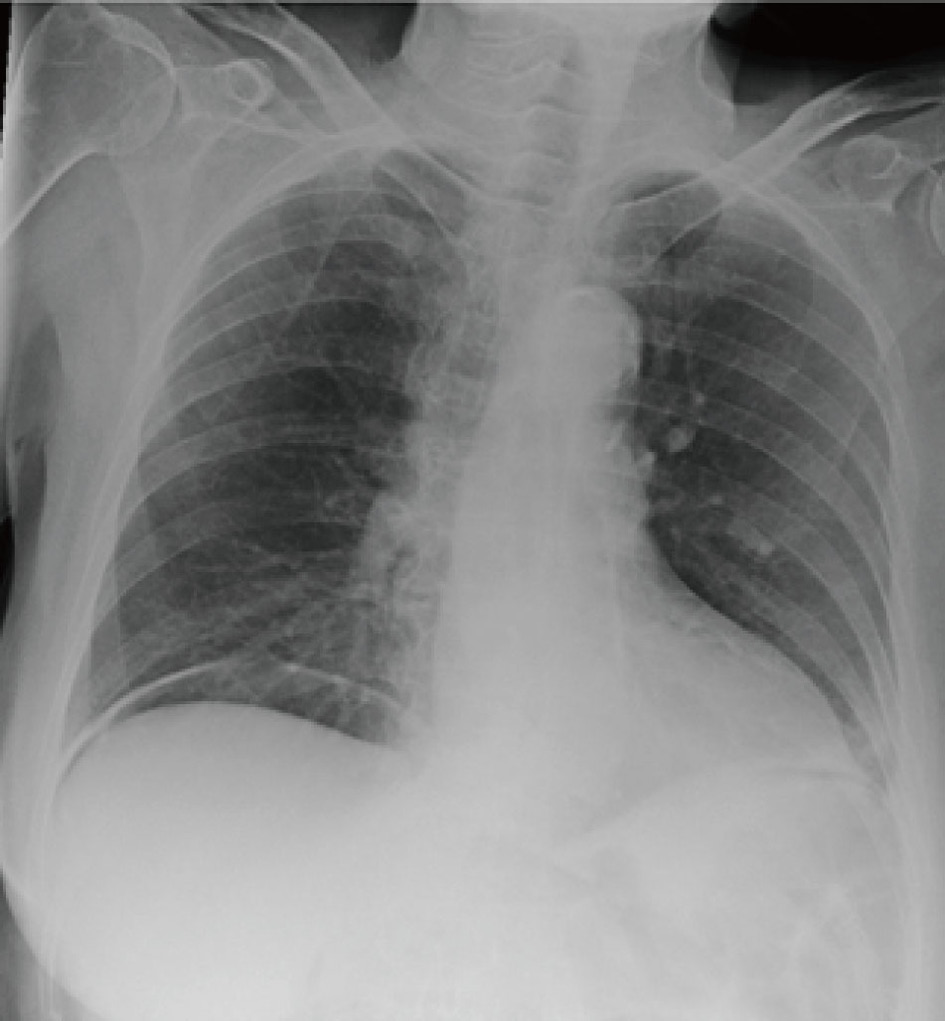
Erect chest x-ray demonstrating free gas under the right and left hemi-diaphragms.
